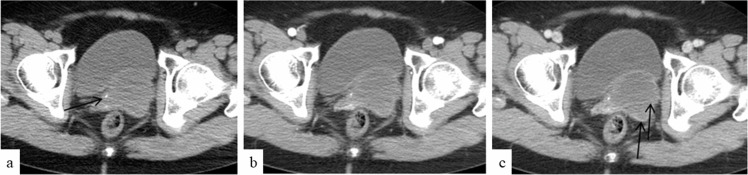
CT image shows vaginal signet ring cell carcinoma. CT image (a) shows the tumor with heterogeneously slightly low density and spotty calcification of the margin (black arrow). Arterial phase image (b) shows mild enhancement of the margin in the tumor. Venous phase image (c) shows further enhancement of the margin and mild grid-like enhancement in the interior of the tumor (black arrows).
